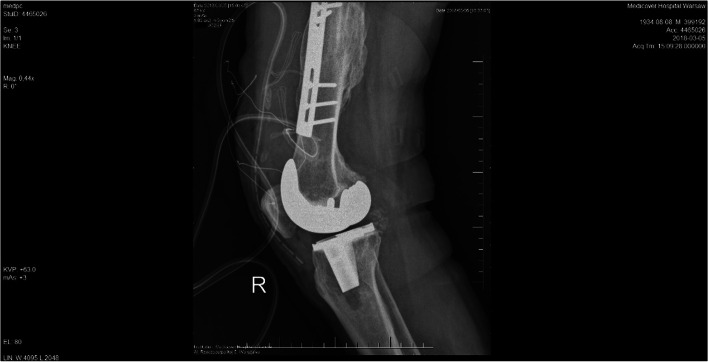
Postoperative X-ray confirming prosthesis placement in the neutral axis
